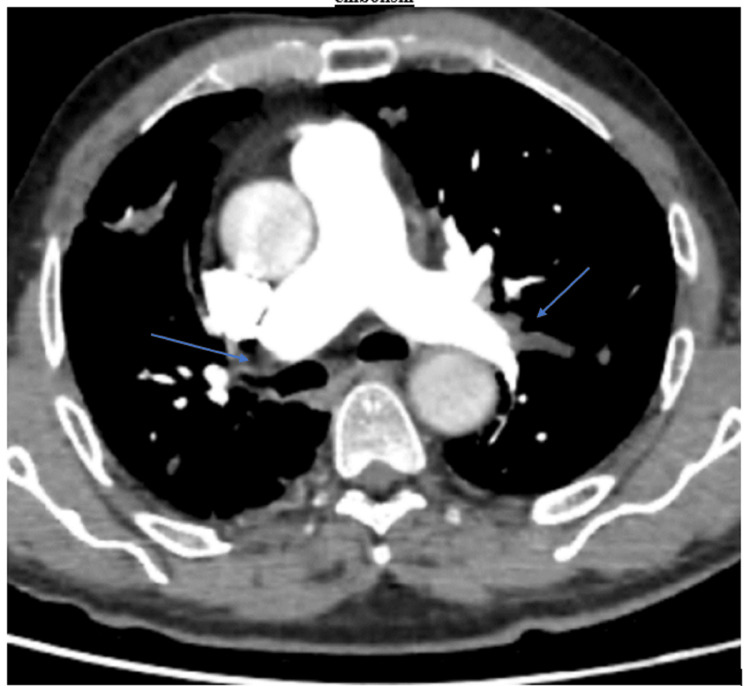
Axial contrast-enhanced chest CT showing bilateral hydatid pulmonary embolism CT: computed tomography
